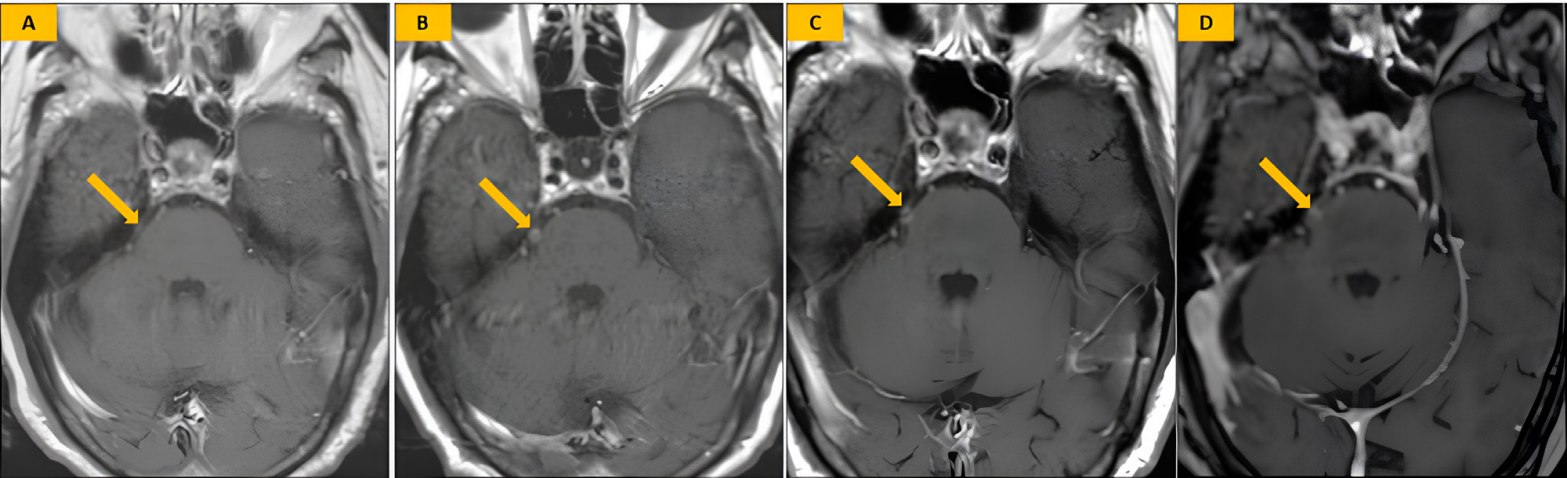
Post-SRS Imaging. (A) Axial T1-weighted contrast-enhanced image at 4 months post-SRS shows no significant imaging changes (*arrow*). (B) Axial T1-weighted contrast-enhanced image at 6 months post-SRS reveals enhancement of the right trigeminal root entry zone (*arrow*). (C) Axial T1-weighted contrast-enhanced image at 12 months post-SRS shows stationary enhancement of the right trigeminal root entry zone (*arrow*). (D) Axial T1-weighted contrast-enhanced image at 18 months post-SRS demonstrates decreased enhancement of the right trigeminal root entry zone along with an emerging focus of encephalomalacia (*arrow*), consistent with expected post-treatment changes. The apparent left-sided enhancement likely represents volume averaging of adjacent petrosal venous structures rather than true pathology, as the patient remained asymptomatic on the contralateral side throughout follow-up.
